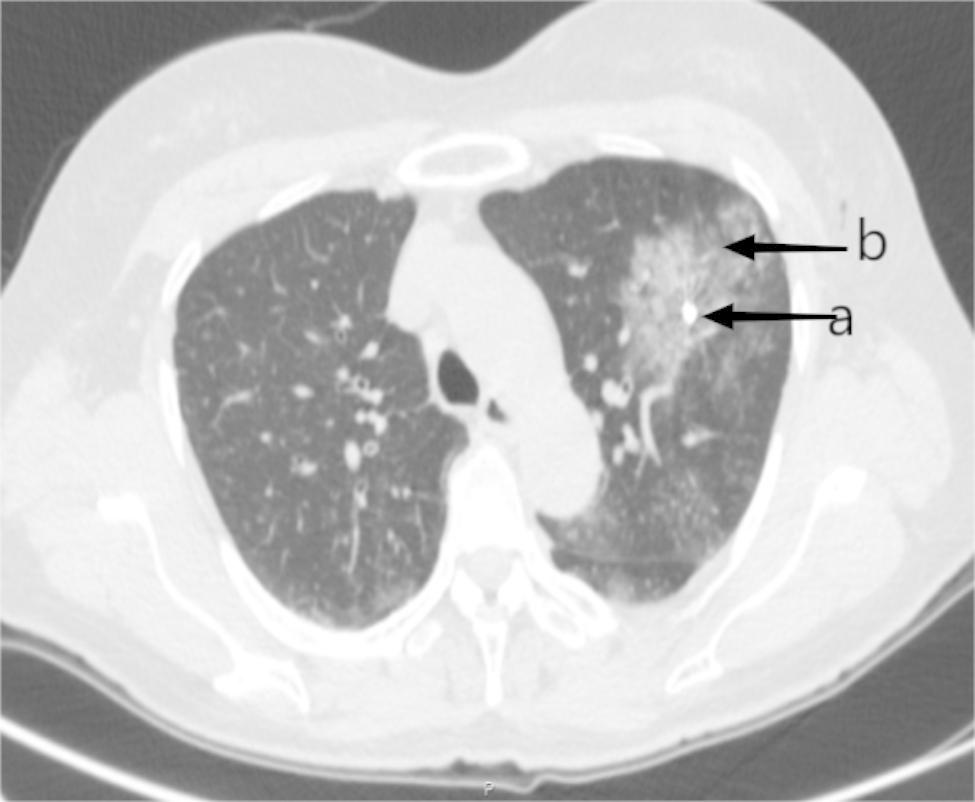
Images of lung CT scan for the development of pulmonary haemorrhage.(a: coil, b: pulmonary hemorrhage)
